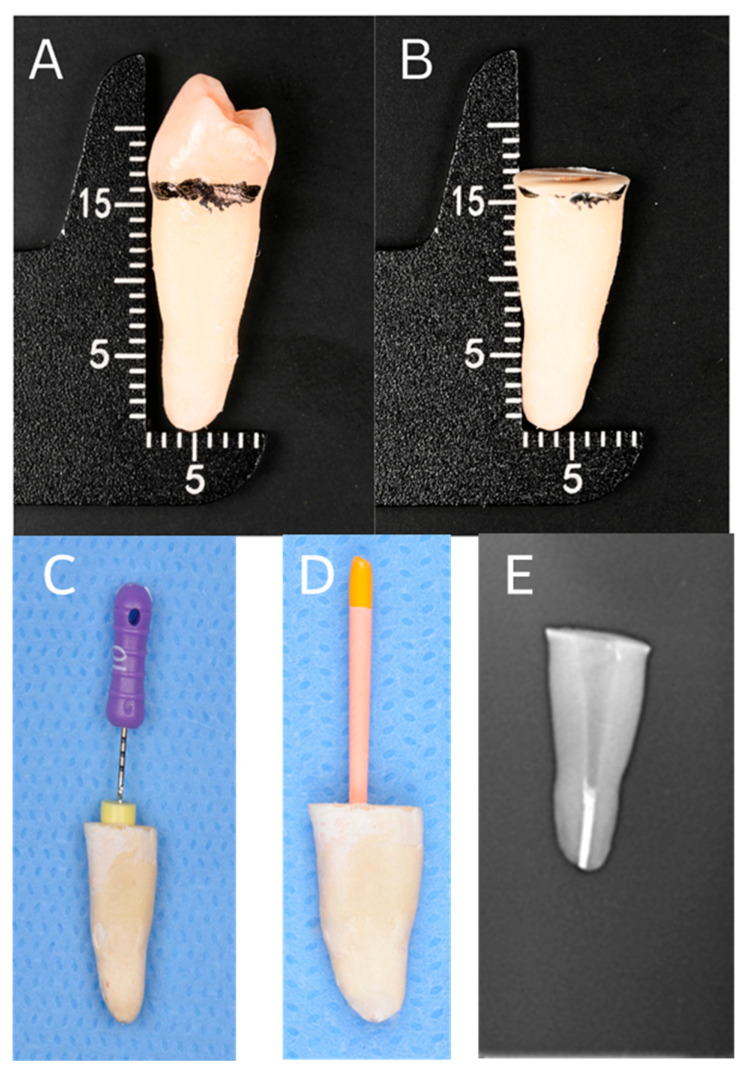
Sample preparation steps. (A): Before the de-coronation, (B): root length after crown de-coronation (16 ± 1 mm), (C): establishment of working length, (D): master cone fitting/obturation, (E): radiograph of obturation.
